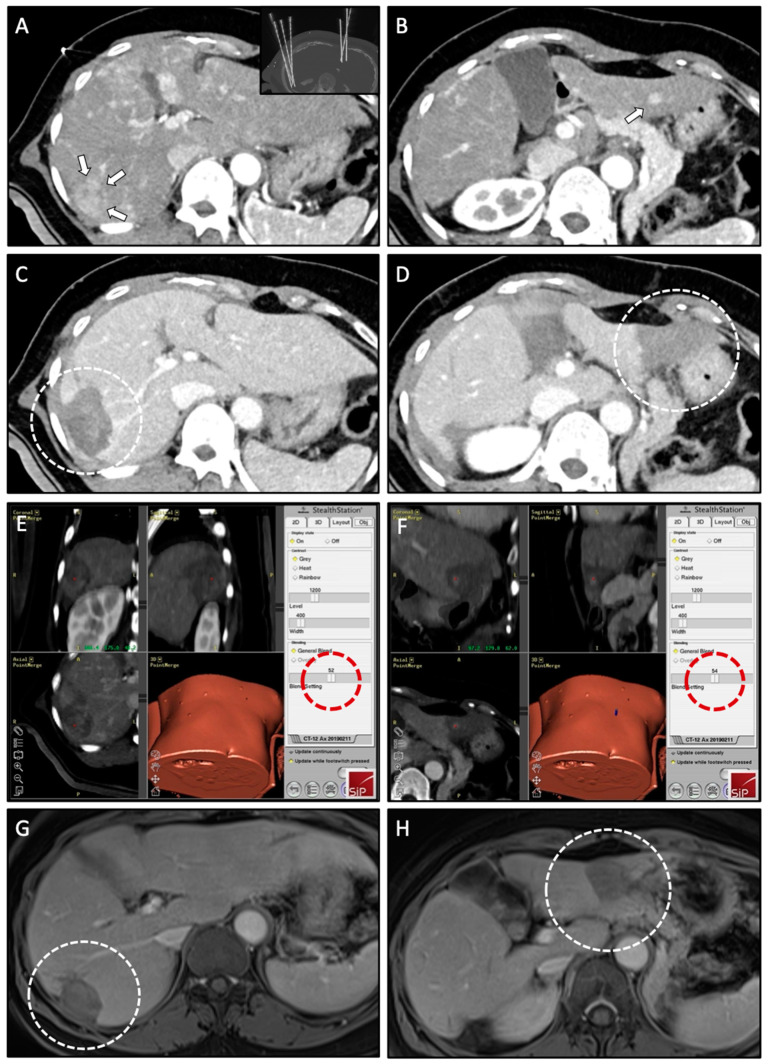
Case of a 57-year-old male with simultaneous treatment of two HCCs (right and left liver lobe) using SRFA. (A,B) Planning CT scan in arterial phase of the SRFA procedure with a 30-mm HCC nodule in liver segment VI (white arrows) and a 9-mm HCC nodule in liver segment III (white arrow); Maximum Intensity Projection (MIP) of the non-enhanced control CT scan showing the position of seven coaxial needles in the upper right corner of (A). (C,D) Control CT scan after ablation with corresponding necrosis zones in liver segment VI and III (white dashed circles). (E,F) Three-dimensional views of the intraprocedural image fusion of the contrast-enhanced planning and final control CT scan using the rigid-registration tool from the navigation system with the possibility to switch between images using the blending function (red dashed circle); verification of complete necrosis including a sufficient safety margin. (G,H) Follow-up MRI scan 18 months after SRFA, showing no evidence of LTP with shrinking necrosis zones (white dashed circle).
